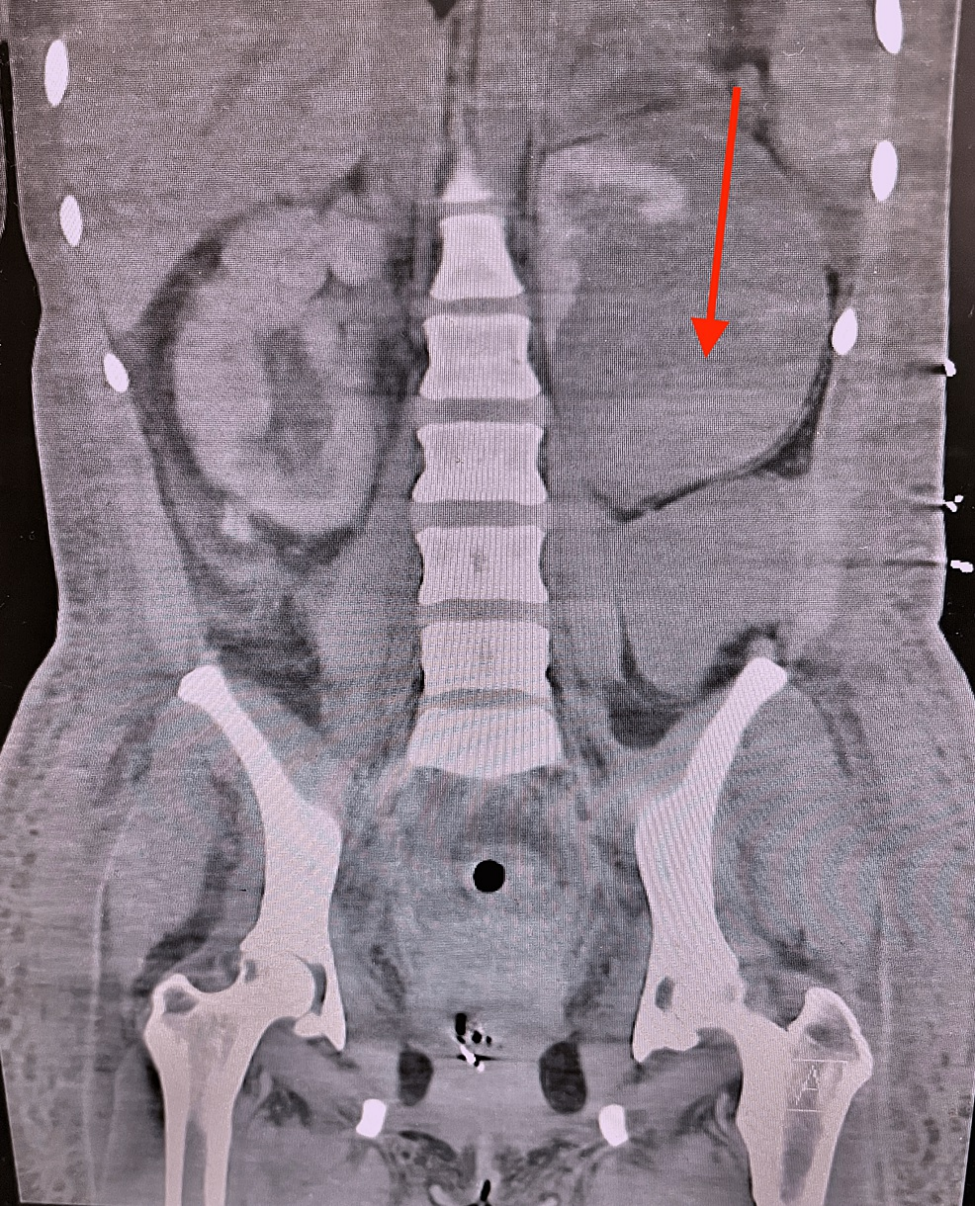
Computed tomography angiography of the chest, abdomen, and pelvis showed a stable-sized renal hematoma, as shown by the red arrow.
